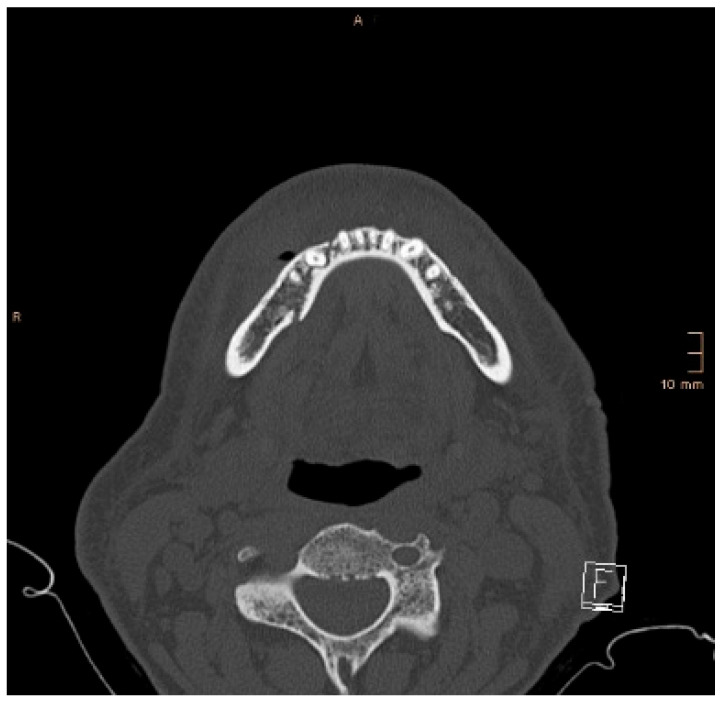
Axial CT scan showing the same patient as in Figure 8. A lingual gap is present after ORIF of the mandibular body fracture, contributing to the inability to reduce the condylar fracture correctly.
